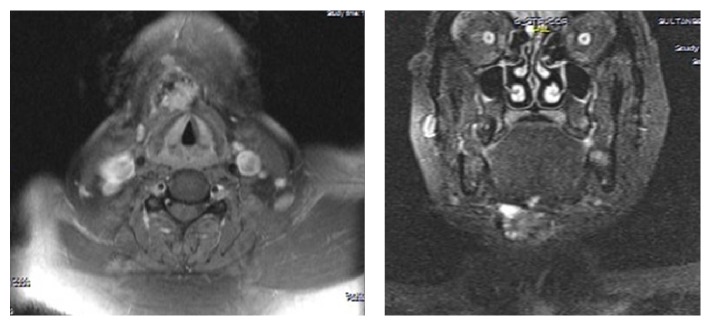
Axial and coronal section MRI demonstrating a solid soft tissue mass containing high signal hyperintensity, cystic changes, and invasion of the infrahyoid muscles.
